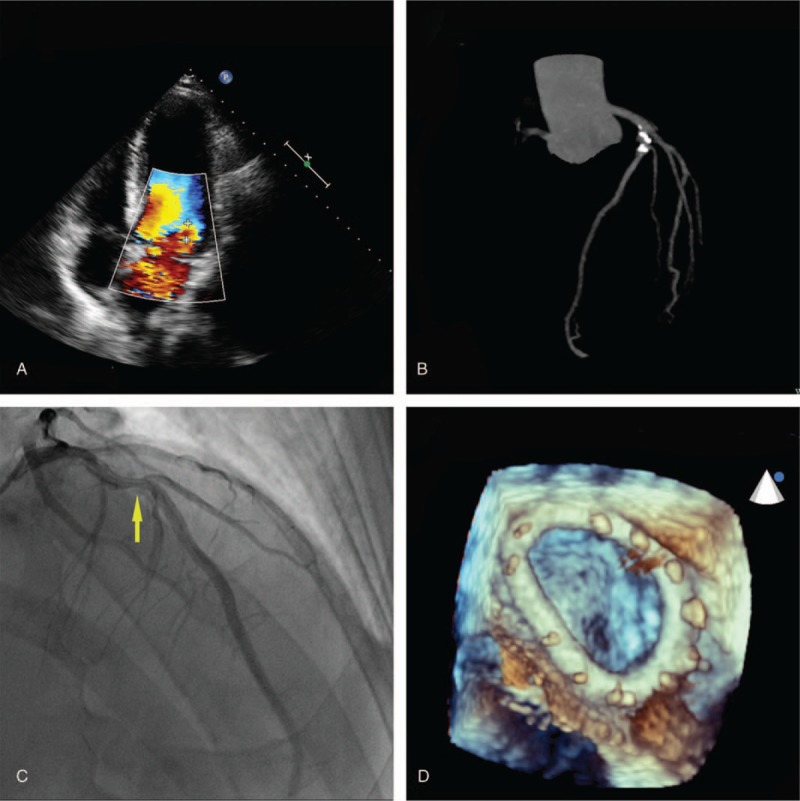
(A) Transthoracic echocardiographyshowed a posterior leaflet prolapse of mitral valve with moderate to severe regurgitation. (B) Coronary computed tomography angiography demonstrated calcification and moderate stenosis in the left anterior descending coronary artery (LAD). (C)Coronary angiography showed a 60% stenosis in the proximal LAD (yellow arrow). (D) Three-dimensional imaging of transesophageal echocardiography revealed no regurgitation after mitral valve repair with the use of an annuloplasty ring.
